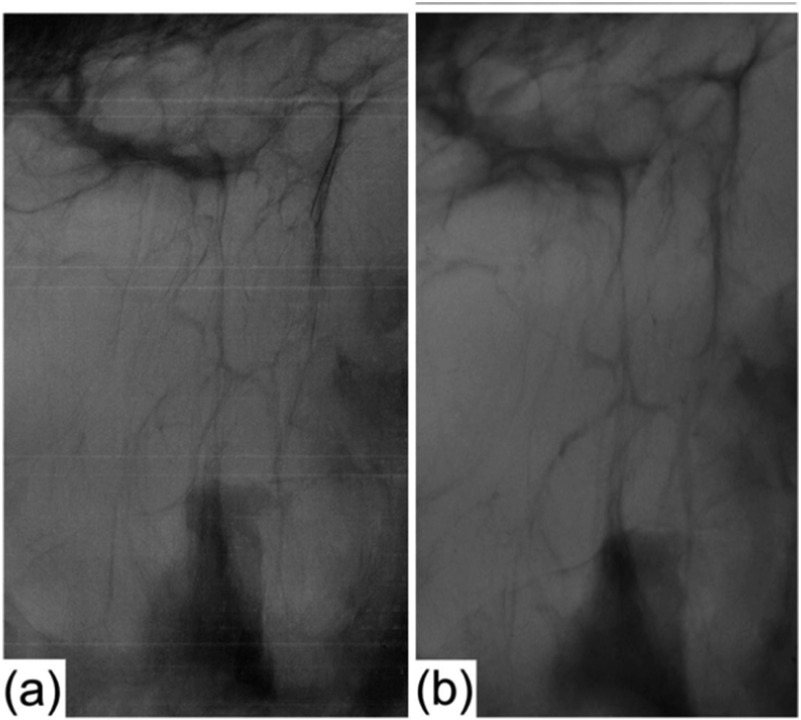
Stomal trabeculae in breast tissue (along which tumours tends to spread) visualised by EI XPCI (a) and conventional x-rays (b).
